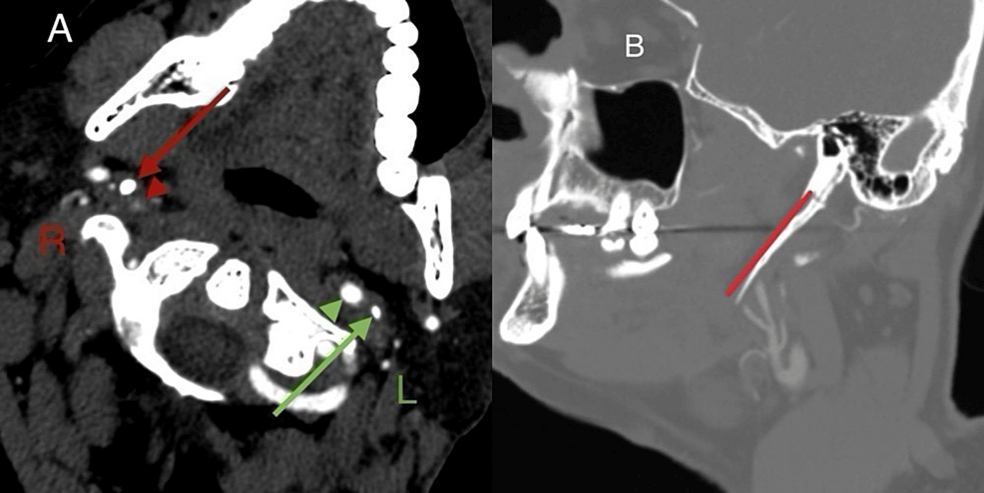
CT showing elongated styloid process in close proximity to right ICA (A) On right side, red arrow is showing thick styloid process adjacent to thrombosed ICA (red arrow head),  as compared to the left side where green arrow is showing thin styloid process adjacent to normal ICA (green arrow head); (B) Red line showing elongated styloid process (length=4.53cm). ICA: internal carotid artery
